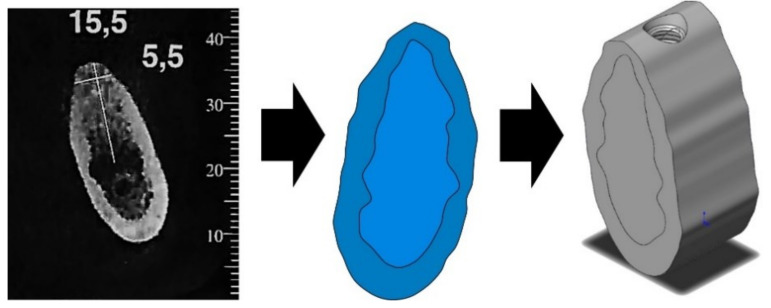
Left: CT scan. Center: mandible section obtained from CT scan. Right: isometric view of mandible’s model.
